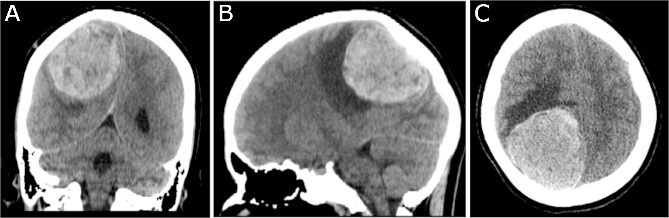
Preoperative CT imaging; (A) coronal, (B) sagittal, and (C) axial CT scans show a large right parietooccipital lesion with vasogenic oedema, mass effect, and midline shift.
